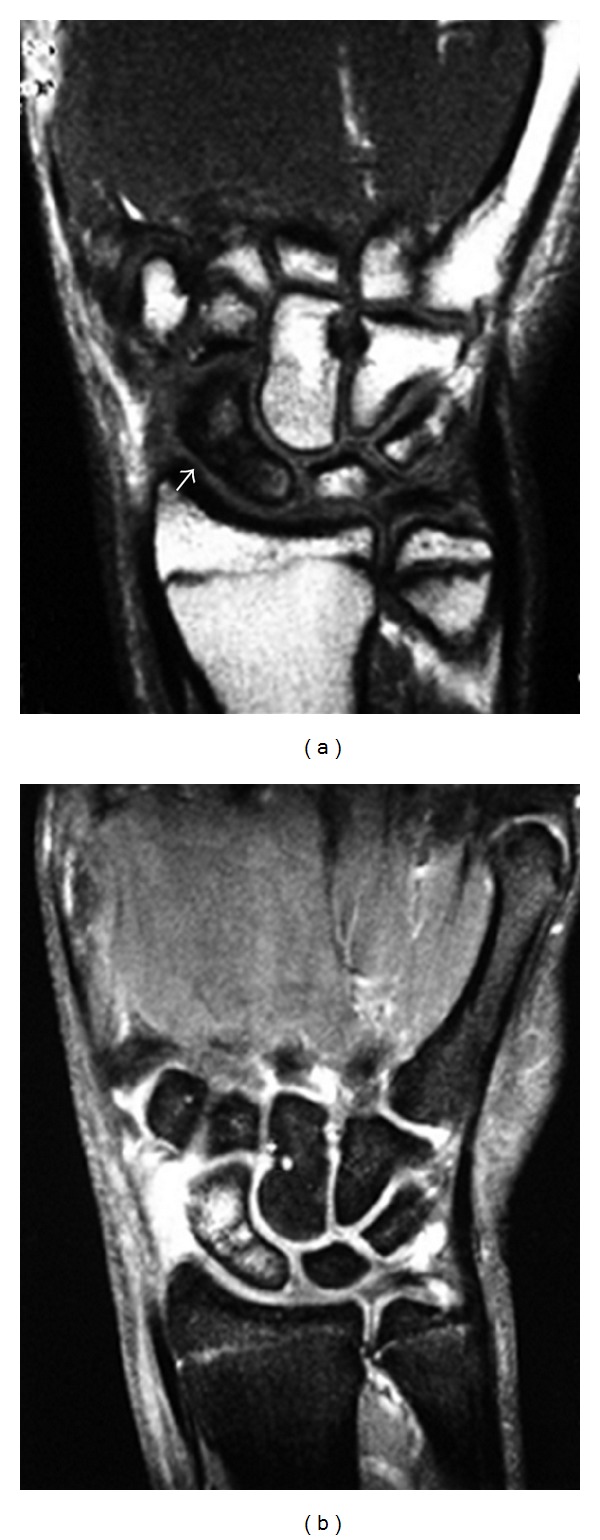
(a) T1-weighted coronal magnetic resonance image showing incomplete fracture at the waist of the scaphoid (white arrow); (b) T2-weighted coronal magnetic resonance image showing bone edema of the proximal and distal poles.
